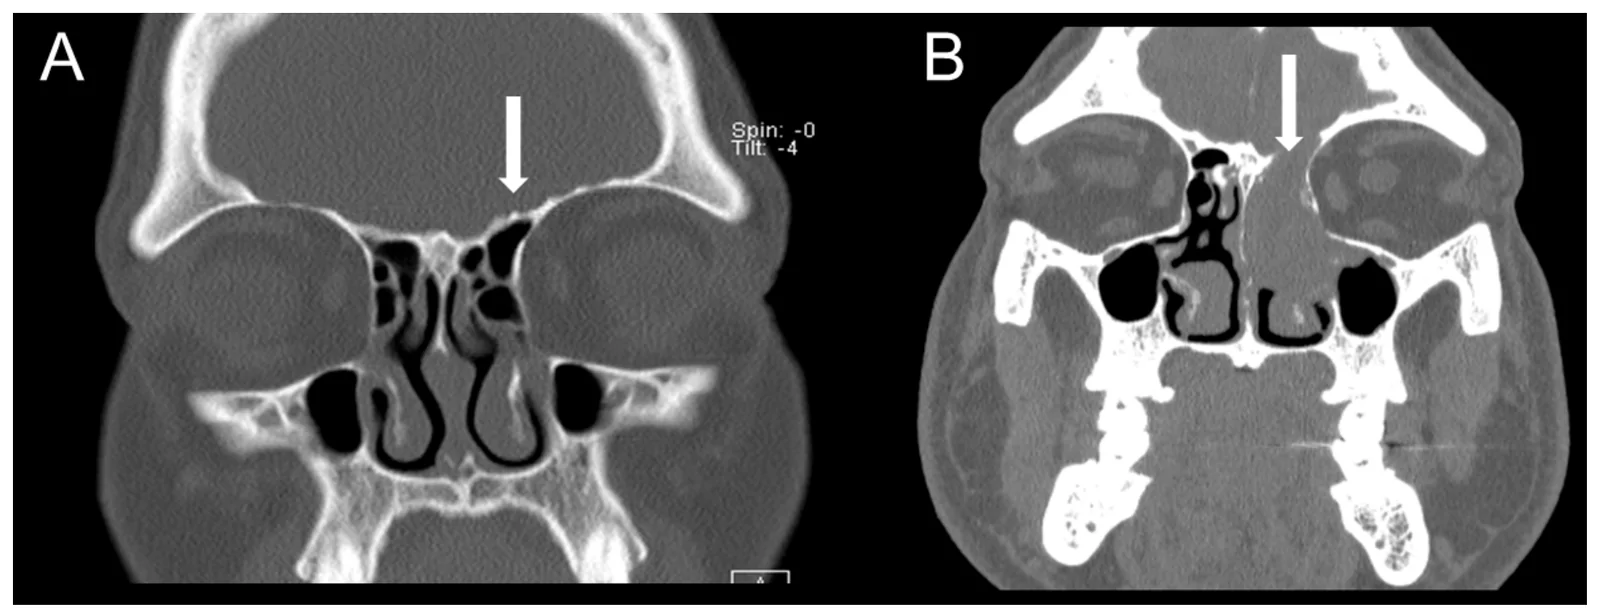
Coronal CT scans from a patient with undiagnosed IIH who underwent endoscopic CSF leak repair of a small right-sided cribriform defect in 2006 (A) and later presented in 2022 with a large left frontal sinus posterior table defect and encephalocele (arrow) (B), in the setting of untreated IIH.
